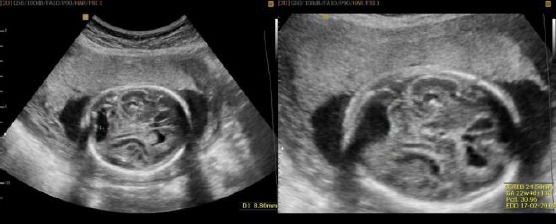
Fetal brain ultrasound scan at 36 weeks gestation
